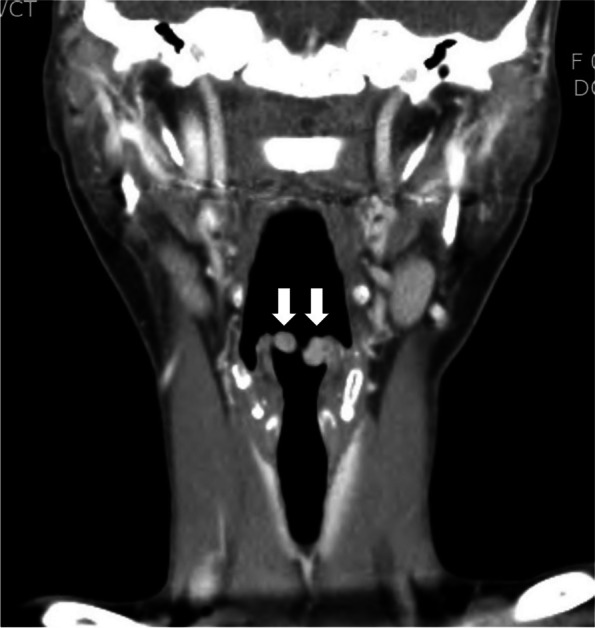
Coronal computed tomography scan of the neck shows bilateral enhancing nodular lesions (arrows) arising from both aryepiglottic folds
